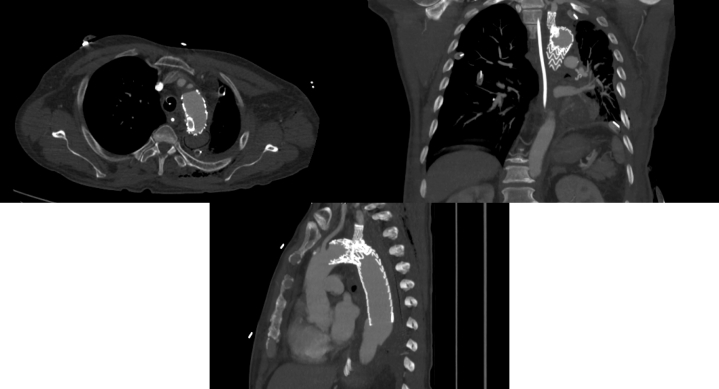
Preoperative Computed Tomography Scans of a Large Perigraft Fluid/Gas Collection
